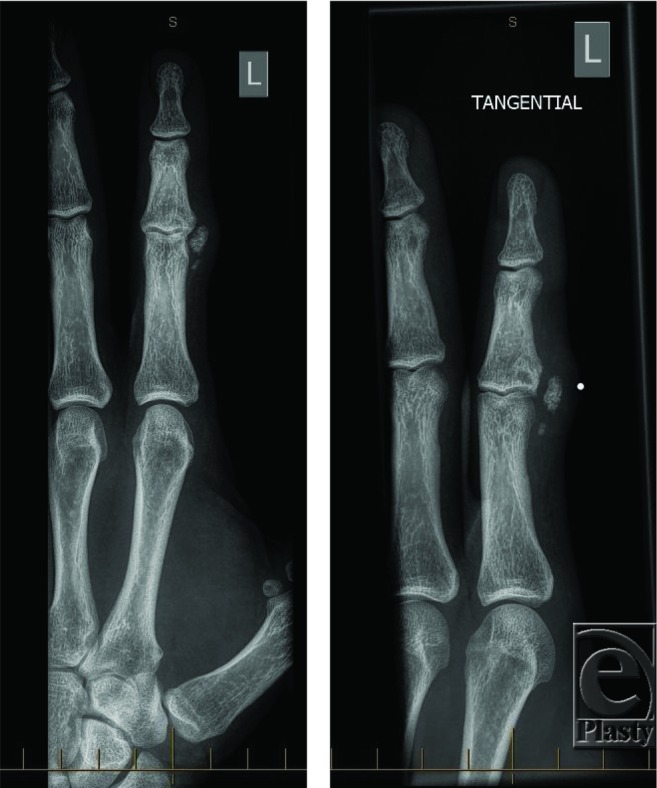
Radiograph at the second recurrence showing a calcified lesion along the volar and radial border of the proximal interphalangeal joint, the larger focus distally measuring up to 8 mm.
